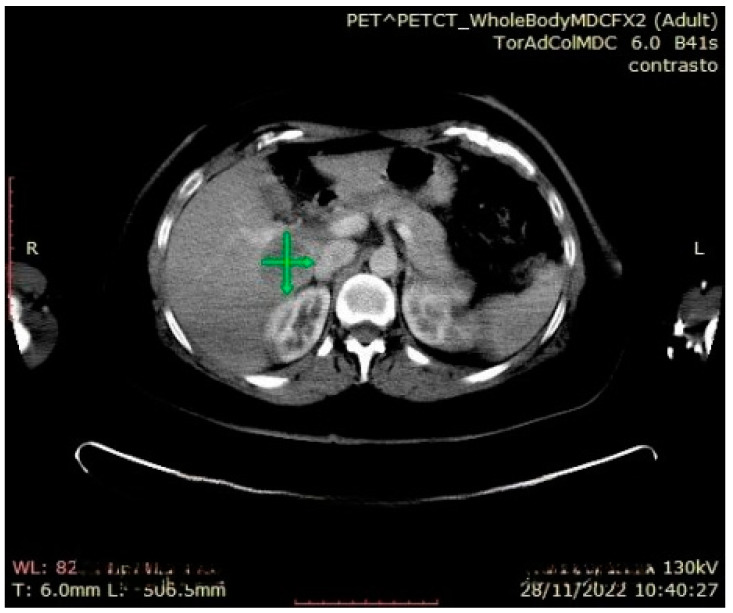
Abdominal CT: hepatic metastasis identified with green arrows.
